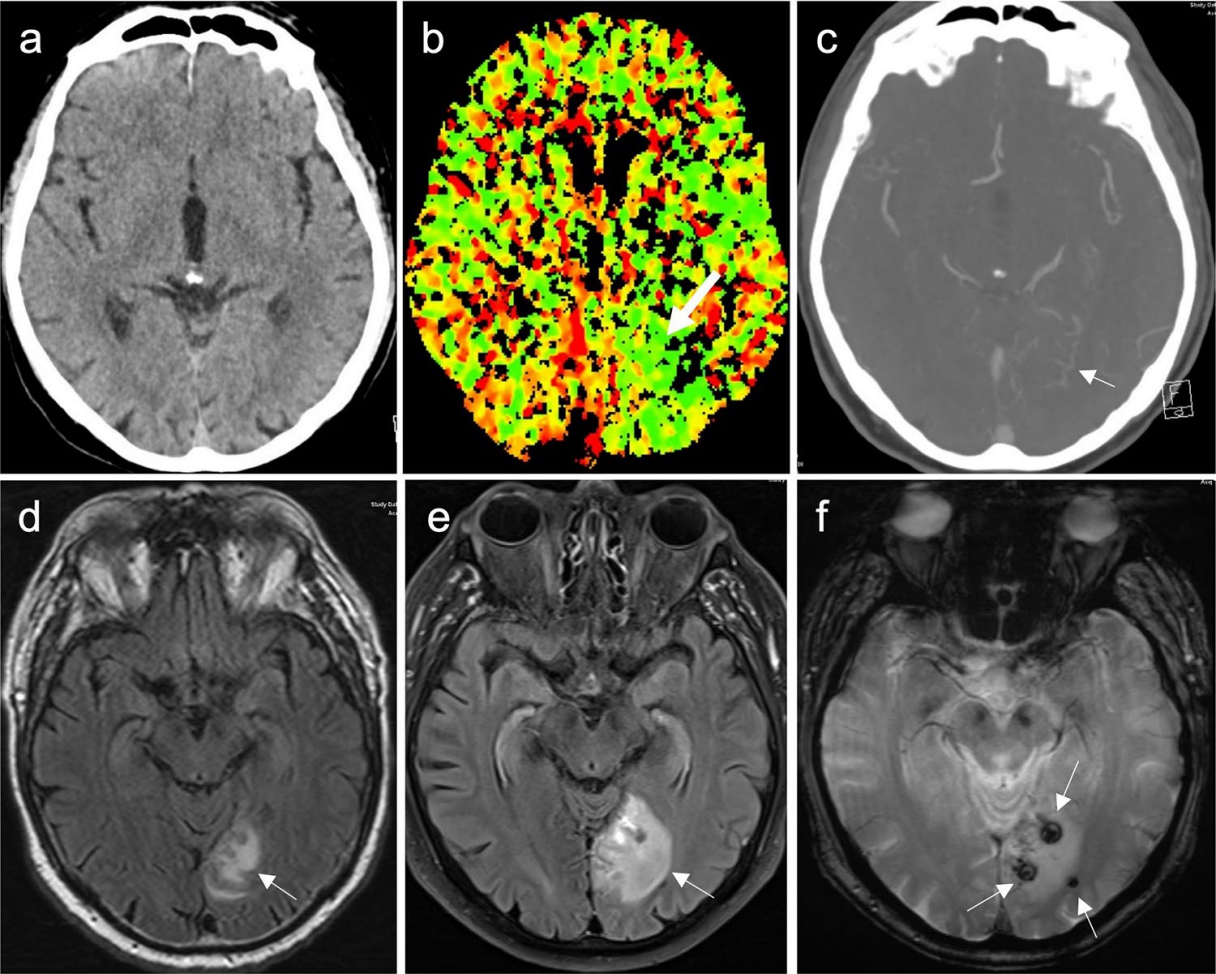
a–c Axial views of the first CT: a native CT, soft tissue window; b corresponding CTP; c corresponding CTA, which shows no hemorrhage or edema occipital. The CTP shows an hyperperfusion of the left occipital lobe, the CTA reveals one tortuous vessel in the described area (all specified findings are marked by an arrow in the corresponding picture). d–f Follow-up-MRIs (five and six days after the first CT): d axial FLAIR with T2-hyperintense edema (arrow); e follow-up axial FLAIR MR image one day after d, with an increasing volume of the edema; f hemosiderin depositions indicate hemorrhages
